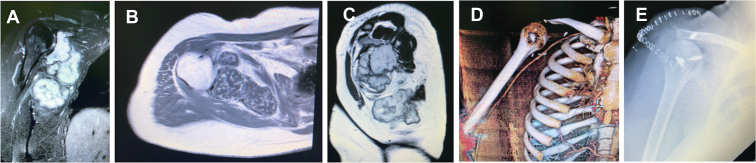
Example of total scapulectomy with suspensoplasty. Tumor invading the entire scapula: frontal MRI section (T2 STIR, A) with hyper signal lesion in the scapula, axial section (T1, B) with lesion of the body of the scapula in hyposignal and sagittal section (T1, C) with hyper signal lesion. Resection of the entire scapula visualized on the 3D CT scan (D) and postoperative radiograph (E) associated with resection of the trapezius, deltoid and posterior superior cuff and suspension with anchors. *MRI*, magnetic resonance imaging; *3D*, 3-dimensional; *CT*, computed tomography.
